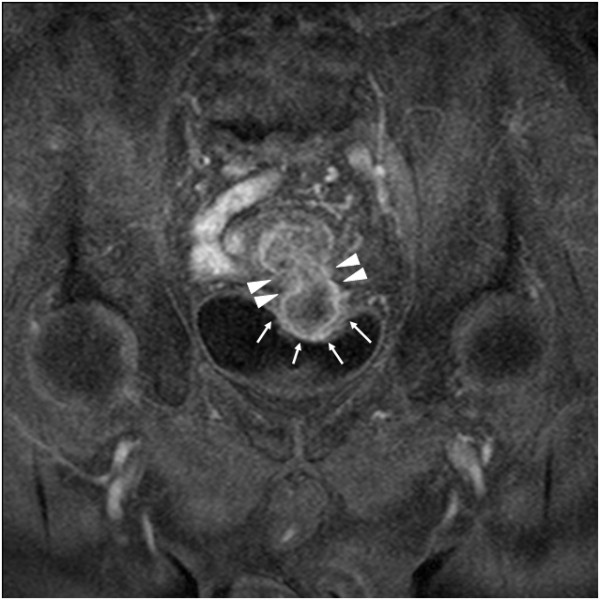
Enhanced MRI T1-weighted image. Between the sigmoid colon and the urinary bladder, the solid tumor was connected via the fistula, showing a dumbbell-shaped appearance. The sigmoid colon tumor protruded into the urinary bladder lumen (arrows) via the colovescal fistula (arrowheads).
